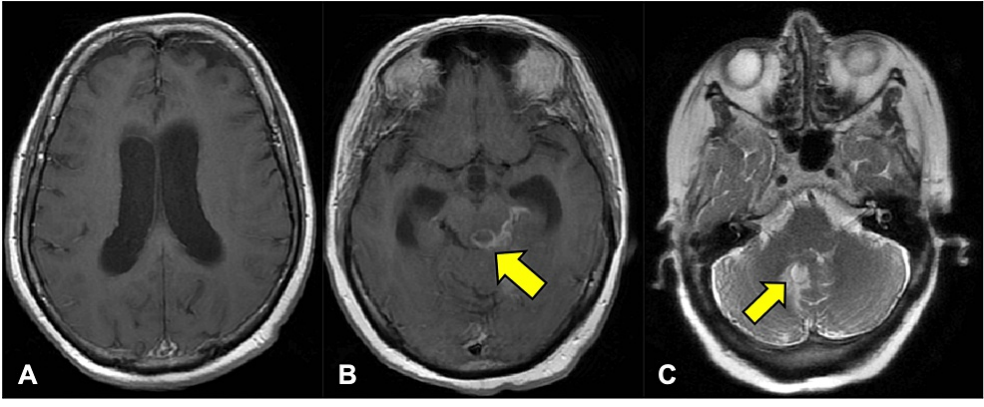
An MRI brain with contrast at the time of presentation demonstrates moderate to severe obstructive hydrocephalus (A) and a small rim-enhancing lesion in the left dorsal midbrain (yellow arrow in B). A subjacent signal abnormality, indicative of perilesional hemorrhage on additional sequences, is partially visualized. MRI brain with contrast five months post-diagnosis (C) shows a cystic focus within the right medial cerebellum, right posterior to the fourth ventricle (yellow arrow) MRI: magnetic resonance imaging
